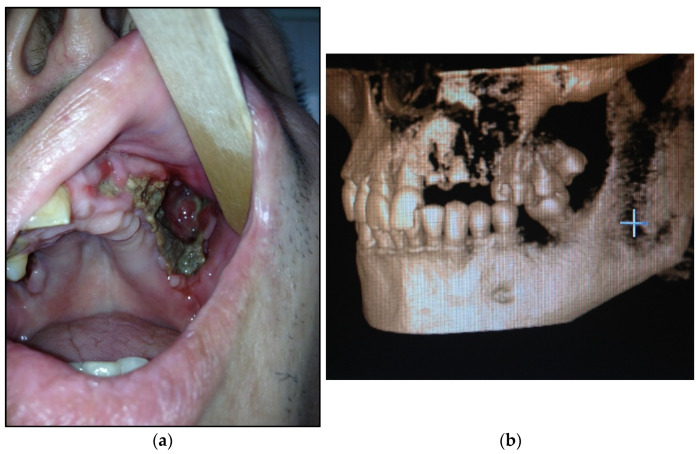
(a) Patient with type 1 toxic phosphorus osteonecrosis. Intraoral view; (b) CT-scan of patient’s skull.
